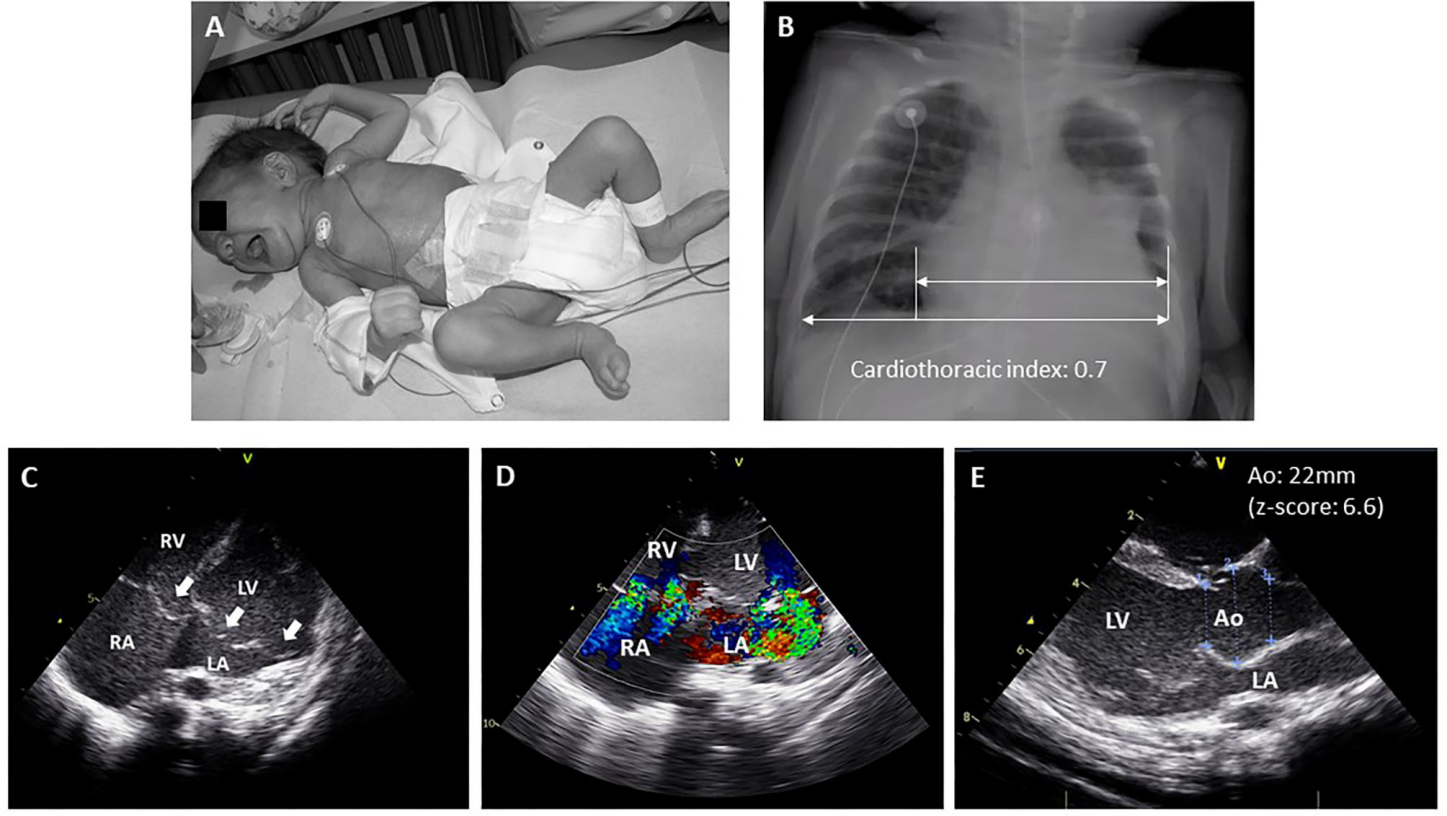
Typical manifestations of neonatal Marfan syndrome. (A) Child with neonatal MFS showing arachnodactyly, long feet, crumpled ears, lipodystrophy and mild pectus excavatum. (B) Chest X-ray showing cardiomegaly and mild scoliosis. (C) Echocardiographic four-chamber view showing mitral and tricuspid valve prolapse (white arrows). (D) Echocardiographic apical four-chamber color doppler view showing moderate-severe mitral and tricuspid valve regurgitation. (E) Echocardiographic parasternal long axis view showing enlargement of the sinus of Valsalva. Ao, aorta; LA, left atrium; LV, left ventricle; RV, right ventricle; RA, right atrium.
